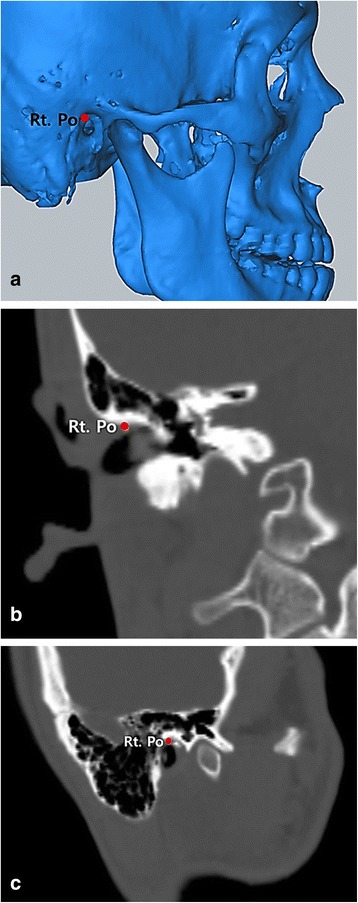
Determination of Po point on 3D CT image. Po was defined as the most superior and outer point on the ovoid bony shadow of the external auditory meatus. a Lateral view of 3D reconstruction image. b Frontal view of right Po. c Lateral view of right Po. *Po* porionEAM: the center point of the most outer round or ovoid shadow of the external auditory meatus, both sides (Fig. 2)
